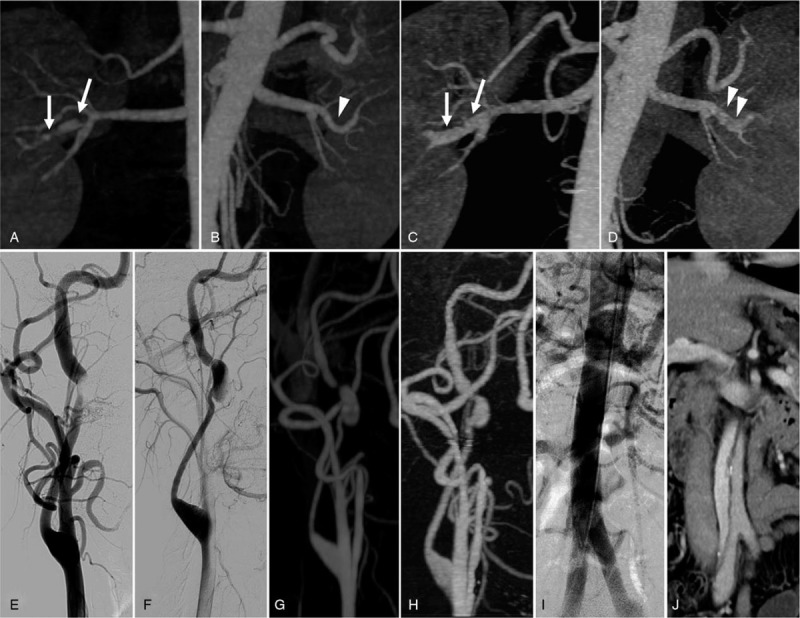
Radiologic findings of symptomatic lesions during the follow-up period. Initially observed multifocal stenosis with post-dilatation in the right renal artery (A, arrows) and normal left renal artery (B, arrowheads). On follow-up renal computed tomography angiography (CTA), the right renal artery showed dilatation (C, arrows), and the left renal artery showed luminal irregularity with slight dilatation (D, arrowheads). Initial cerebral angiography showed a tapered stenosis with abrupt post-dilatation in the mid-cervical internal carotid artery (E) and a dissecting aneurysm was observed on follow-up (F). There was no further interval change observed on follow-up (G, H). Newly developed dissection was noted in the infrarenal abdominal aorta (J), which was previously normal (I) on abdominal CTA.
